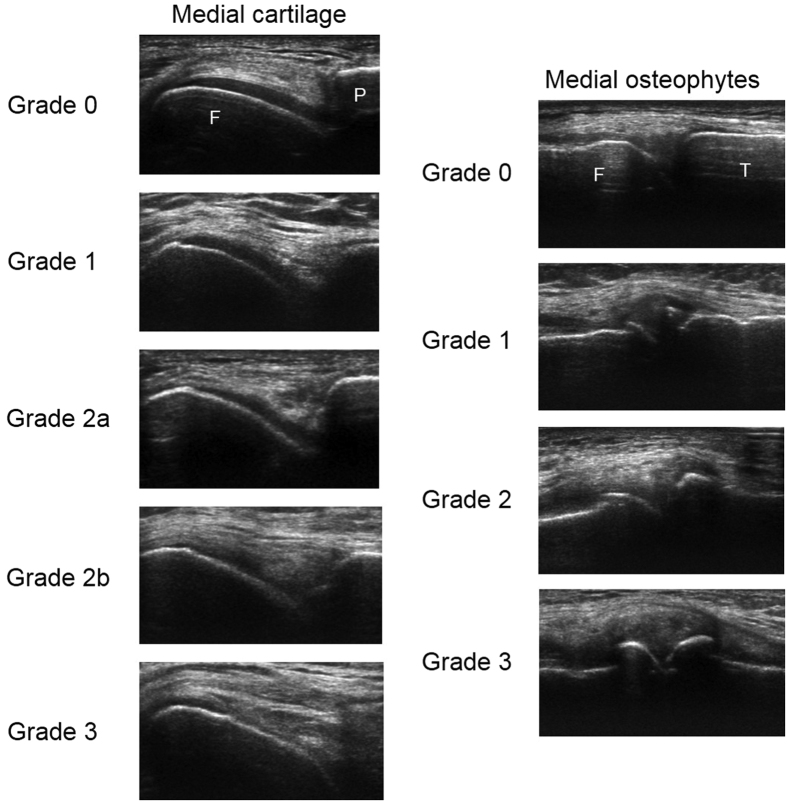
Example ultrasound images for semi-quantitative grading of medial femoral articular cartilage (left, F – femur, P – patella), and medial femoral and lateral osteophytes (right, F – femur, T – tibia). The definitions for articular cartilage degeneration grades^27^ assessed from transversal ultrasound images are as follows: Grade 0 – monotonous anechoic band with sharp hyperechoic anterior and posterior interfaces, Grade 1 – loss of the normal sharpness of cartilage interfaces and/or increased echogenicity of the cartilage, Grade 2a – in addition to the above changes, clear local thinning (less than 50%) of the cartilage, Grade 2b – Local thinning of the cartilage more than 50% but less than 100%, Grade 3–100% local loss of the cartilage tissue. The definitions for osteophyte grades^15^ assessed from longitudinal images are as follows: Grade 0 – no osteophyte, Grade 1 – small osteophyte, Grade 2 – medium osteophyte, Grade 3 – large osteophyte. In these images, the same grade osteophytes are always present on both femoral (F) and tibial (T) margin.
